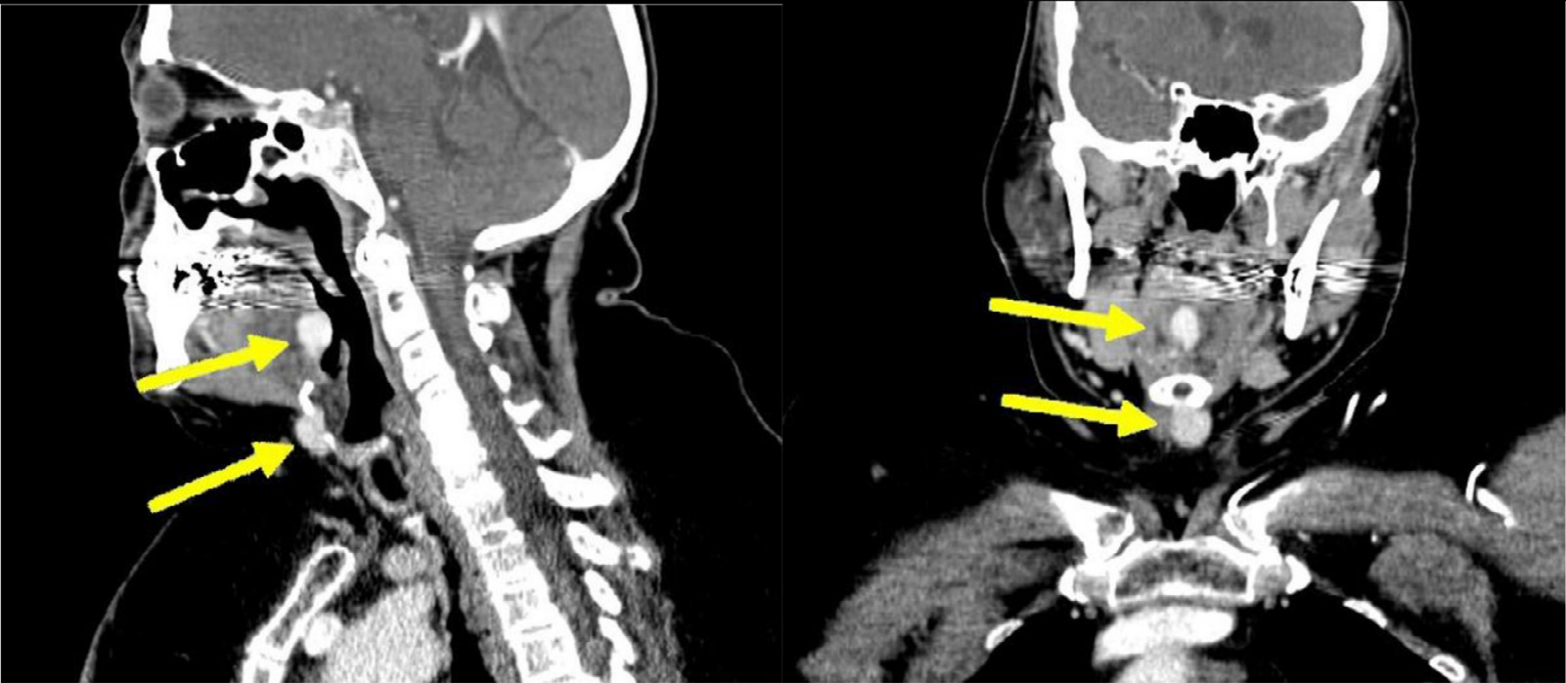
Contrast-enhanced CT of Neck Masses: Sagittal and Coronal images of a contrast-enhanced CT of the neck showing the 2 sites of incidentally noted hyperdense tissue, the more superior of the 2 being at the base of the tongue, the inferior being anterior and adjacent to the hyoid bone.
